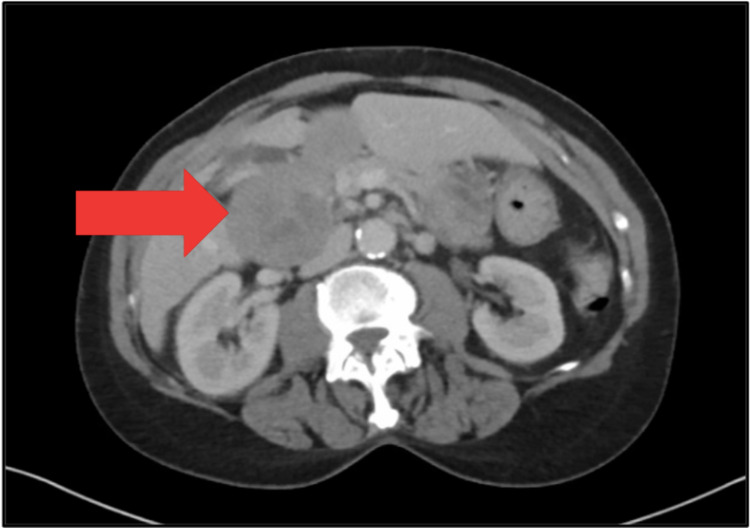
Axial cut of CT abdomen and pelvis showing duodenal cyst.
